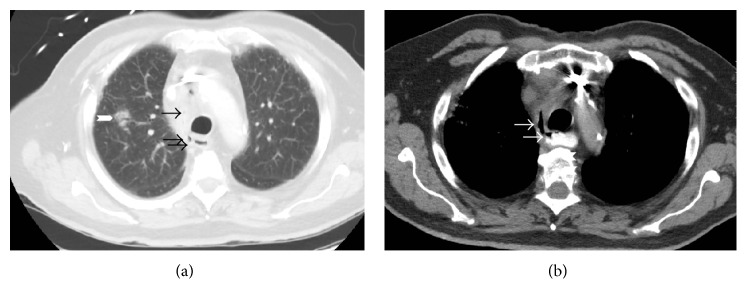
(a) Sagittal CT scan demonstrating fistulous tract containing air bubbles (arrows) and surrounding inflammation extending from the right lateral esophageal wall and right upper lobe pulmonary opacification (chevron). (b) CT esophagram demonstrating fistulous tract (arrows) extending from the right anterolateral esophagus to the mediastinum.
